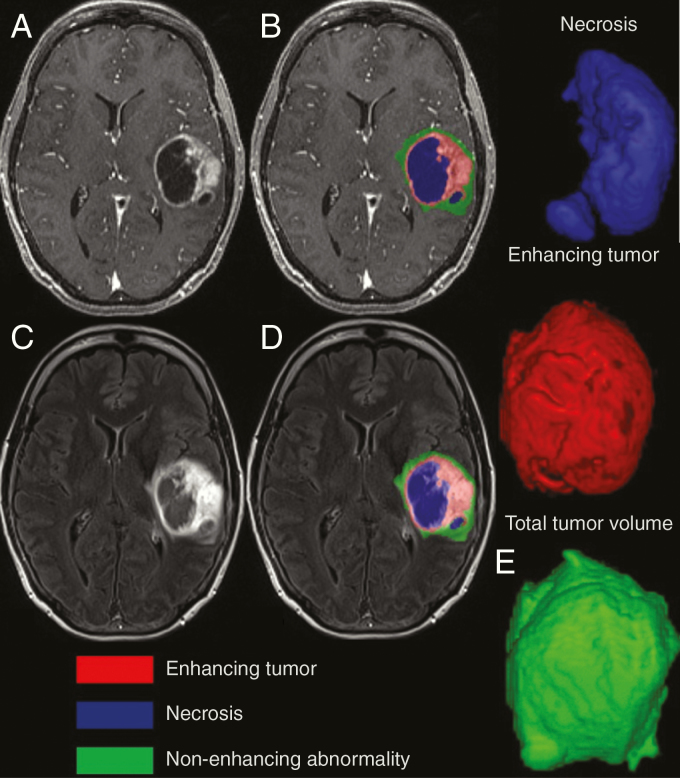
Tumor segmentation in a patient with glioblastoma. Necrosis, enhancing and non-enhancing FLAIR signal abnormality on pre- and post-contrast T1 (A and B) and FLAIR (C and D). (E) The 3D representation of necrosis, enhancing, and total tumor volumes.
